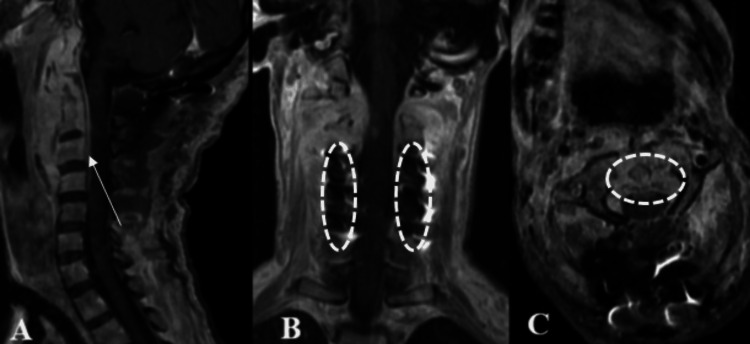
Postoperative T2 MRI A. Sagittal view: Early postoperative MRI following OC fixation shows cervical spine straightening (10°) and decompression at the bulbomedullary junction. Gadolinium enhancement is visible in the retropharyngeal space, indicating postoperative inflammation. Partial reduction of the extramedullary epidural mass is noted. B. Coronal view: Paravertebral hyperintensities are seen at the C2 and C3 levels adjacent to the fixation hardware. C. Axial view at C1 level: The odontoid process appears ventrally repositioned. Hyperintensity surrounds the odontoid and anterior arch of C1, consistent with residual edema and decompression effects.
